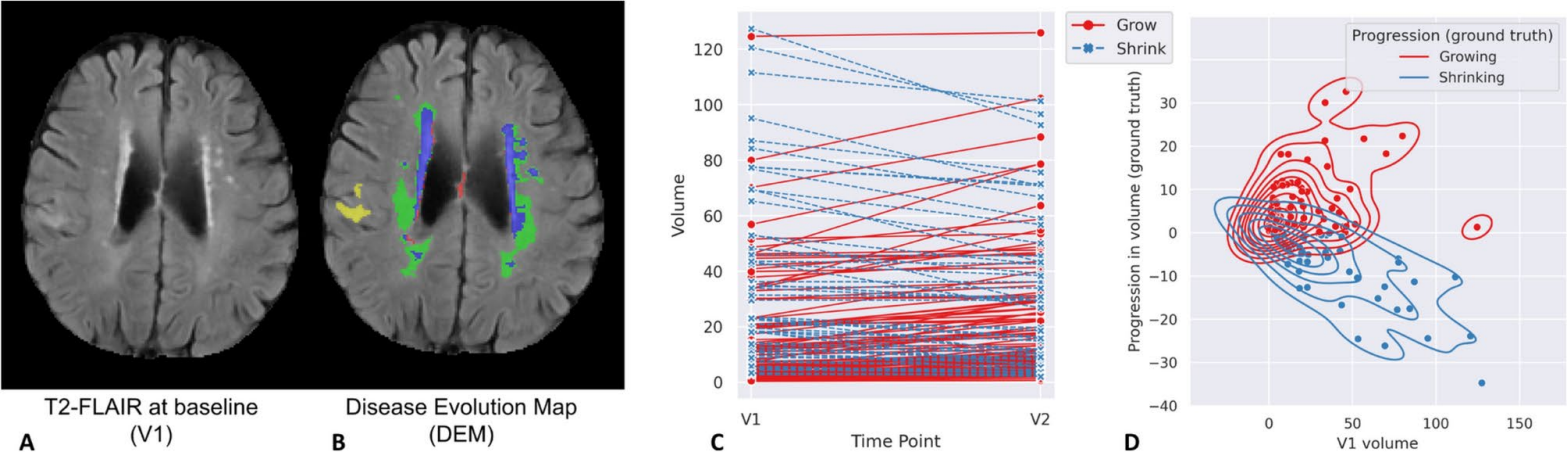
(A): Brain-extracted FLAIR axial slice of the baseline scan or V1. (B): Visualisation of disease evolution map (DEM) of white matter hyperintesities (WMH). Red represents shrinking WMH, green represents growing WMH, blue represents stable WMH, and yellow represents stroke lesions. (C): Volumetric progression of WMH (in ml) from V1 to V2 (1 year apart) for all subjects from our dataset. (D): shows the distribution of volumetric progression of WMH (in ml) based on WMH volume at V1 for all subjects.
